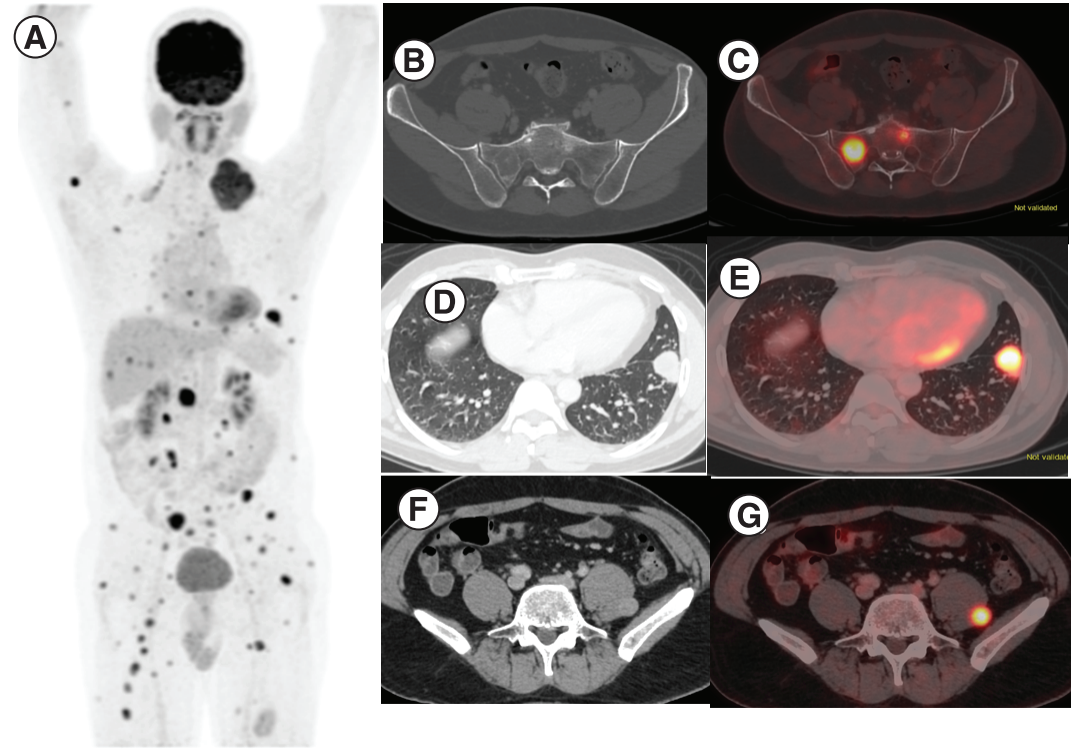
Pre- and post-treatment images of patient no. 9. (A) Pre-treatment axial contrast-enhanced computed tomography thorax showing soft-tissue density in left upper lobe apico-posterior segment. (B) Corresponding fused positron emission tomography-computed tomography image showing increased tracer uptake suggesting lung metastasis. (C & D) Represent post-therapy changes in same patient with complete resolution of metastasis with fibro reticular changes s/o complete response with residual drug toxicity changes.
